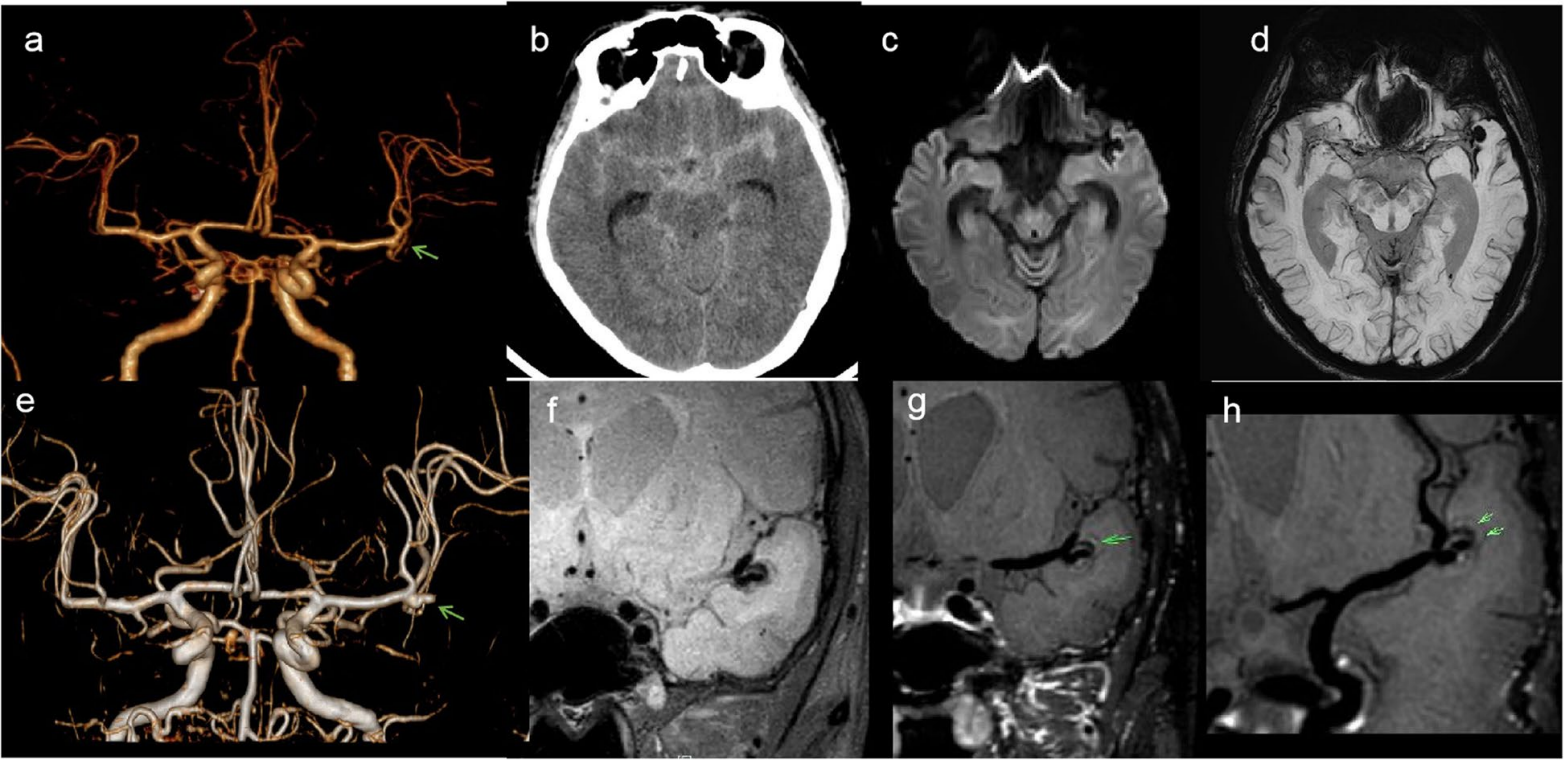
Images of a 48-year-old male patient who presented with transient loss of consciousness and acute headache for 6 h. a Computed tomography angiography (CTA) showed that the M1 segment was slightly swollen at the end, which was not obvious (see arrow). This patient was diagnosed as normal at the time of admission. b Computed tomography (CT) showing the subarachnoid hemorrhage of the patient; c-d 1 month later, diffusion-weighted imaging (DWI) and susceptibility weighted imaging (SWI) showing saccular aneurysm; e magnetic resonance angiography (MRA) showing an aneurysm at the M1 segment (see arrow); and f-h high-resolution magnetic resonance imaging (HRMR-VWI) showing aneurysmal protrusion with thickened residual wall at the end of the M1 segment and a crescent-shaped hematoma under the tunica adventitia (see arrow)
